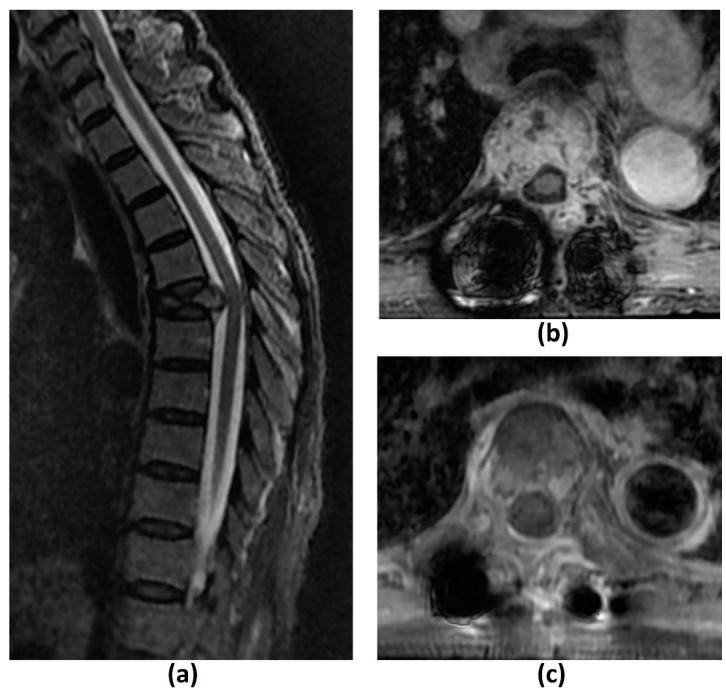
Sagittal T2-weighted image (a) of a 69-year-old female patient with metastatic rectal cancer to the spine shows a pathological T5 vertebral fracture. Axial post-contrast fat-suppressed T1-weighted images of the same patient, post separation surgery and planning for stereotactic body radiotherapy (SBRT) (b), and 6 months post-treatment (c). There is reduced tumour enhancement and bulk between (b) and (c) due to favourable post-SBRT response.
